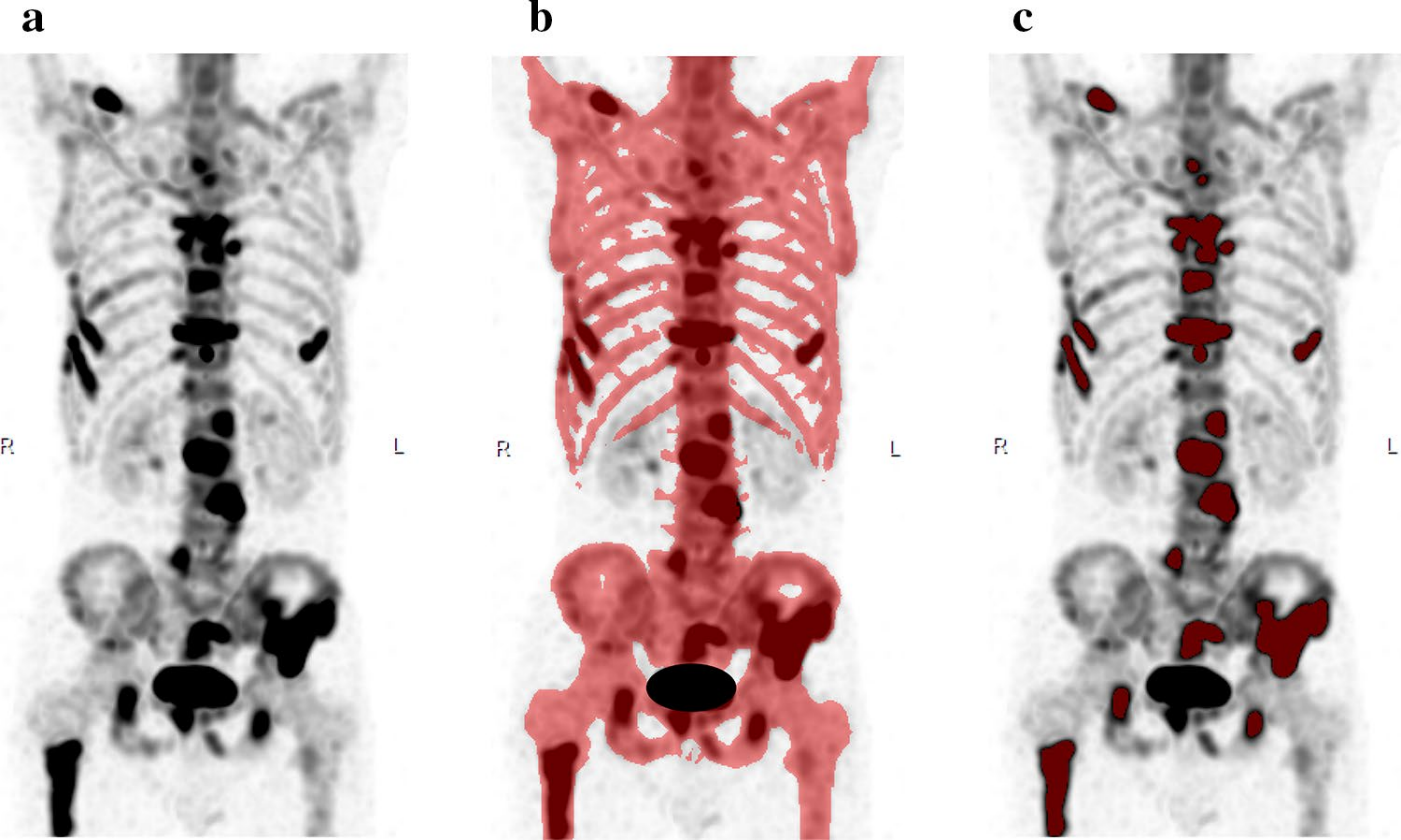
The process of determining total bone uptake (TBU). A maximum intensity projection (MIP) image was selected to explain. MIP image of the trunk was obtained by bone SPECT/CT study (a). Bone image (red) was obtained by subtracting non-bone components using 152 CT Hounsfield units (b). Areas above SUV = 7.0 was considered as the active bone metastatic burden (red in c). Then, TBU was obtained as the sum of (mean SUV above SUV = 7.0) × (volume above SUV = 7.0) for each lesion
